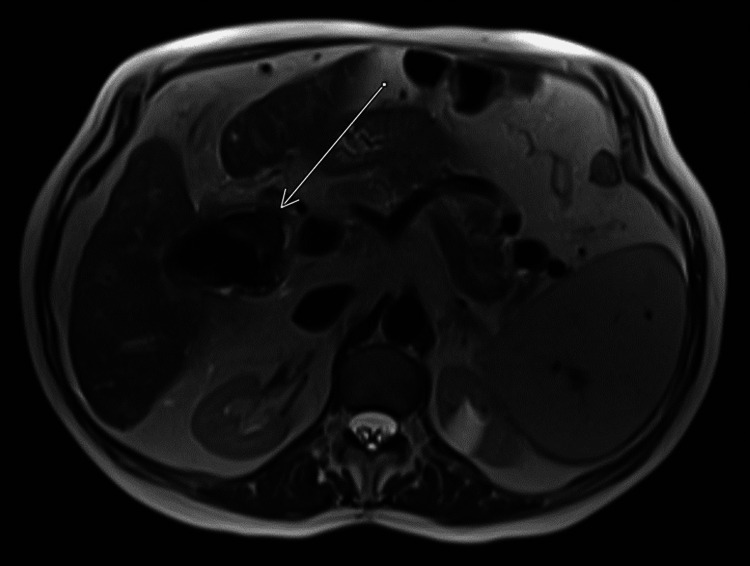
MRI demonstrating indistinct wall between the gallbladder neck and the common bile duct concerning for pneumobilia (arrow) MRI: magnetic resonance imaging
